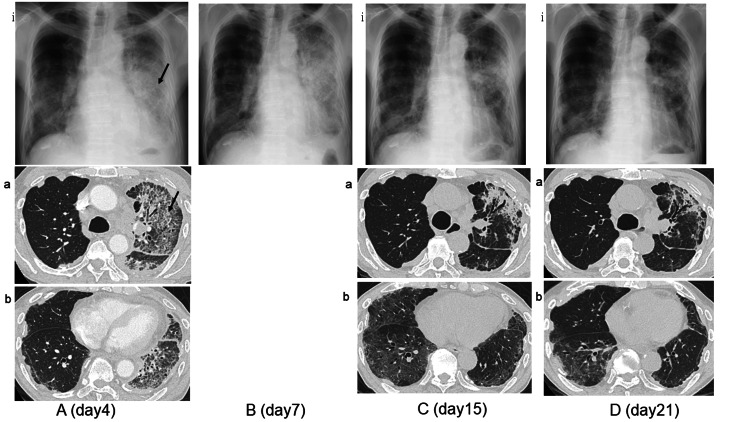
Radiological course after pembrolizumab administration. A. On day 4 after pembrolizumab administration, chest X-ray (ⅰ) and chest CT (a, b) revealed a widespread consolidation and ground-glass opacity on the same side as pleurodesis (black arrows). B. On day 7, the lung shadows apparently worsened. C. On day 15, the left lung shadow was dramatically improved whereas chest X-ray (ⅰ) and chest CT (a, b) showed the emergence of ground-glass opacity (GGO) in the right lower lobe. D. On day 21, chest X-ray (ⅰ) and chest CT (a, b) showed the bilateral shadows improved.
